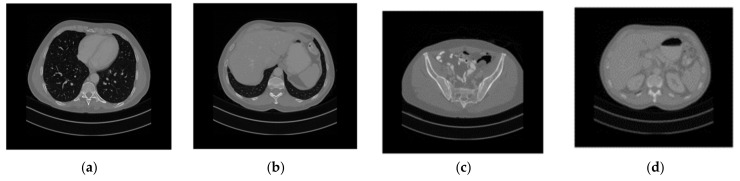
Plain medical CT images. (a) Original “001” image, (b) original “002” image, (c) original “003” image, (d) original “004” image.
